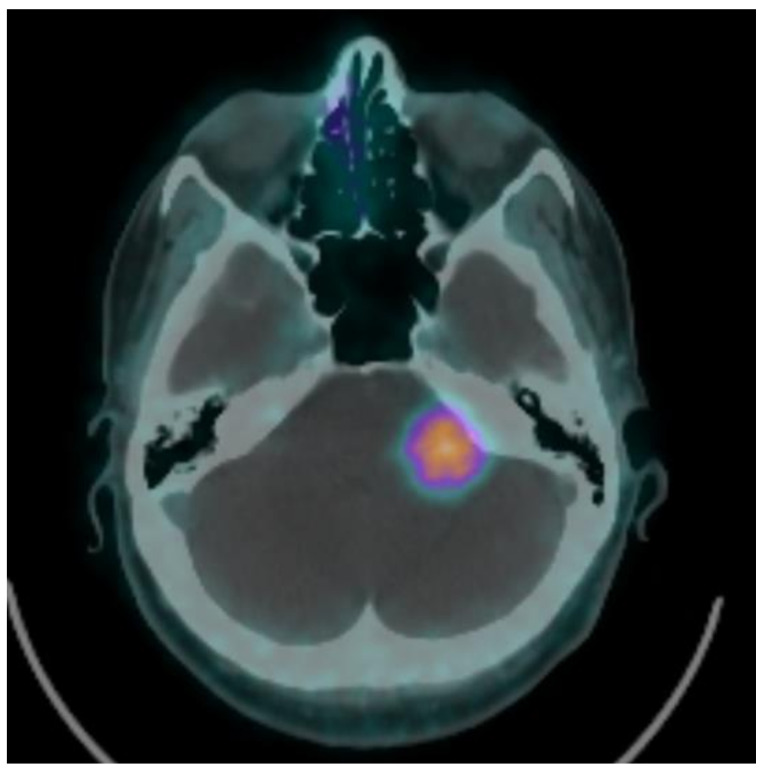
^68^Ga-DOTATOC positron emission tomography (PET)/CT allows differential diagnosis with caustic schwannoma. Standardized uptake value (SUV): 7.1.
